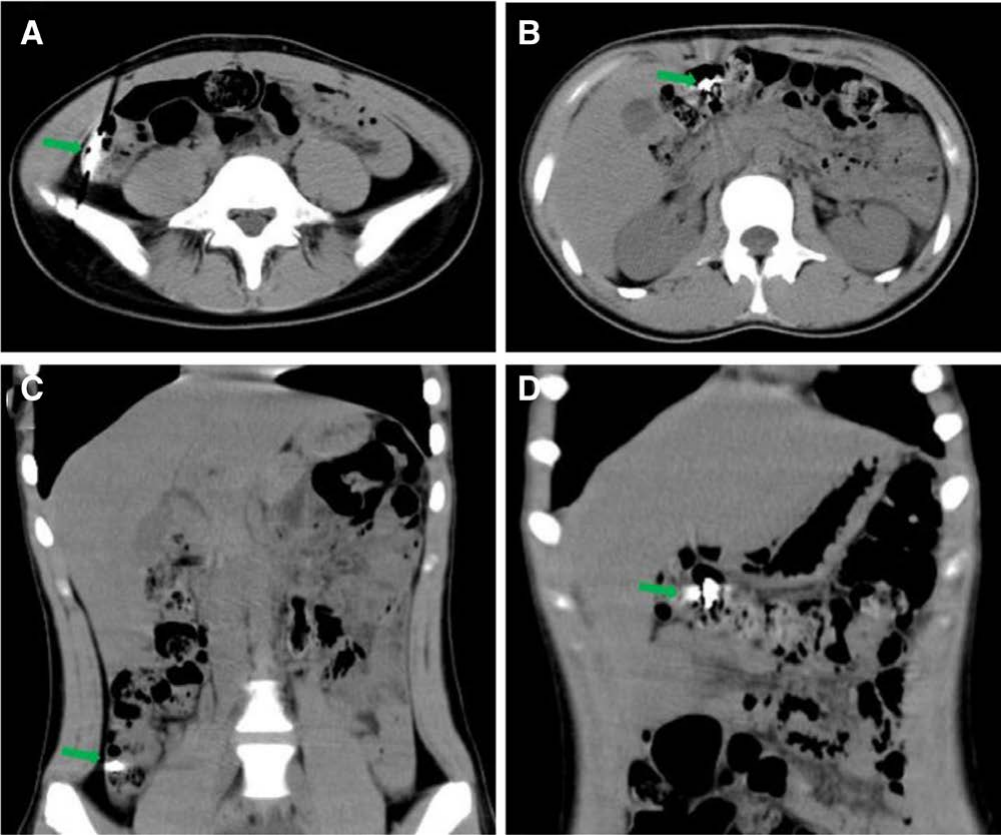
Abdominal CT showed 2 dense shadows in the ascending and transverse colon (A–D, green arrow). CT = computerized tomography.
